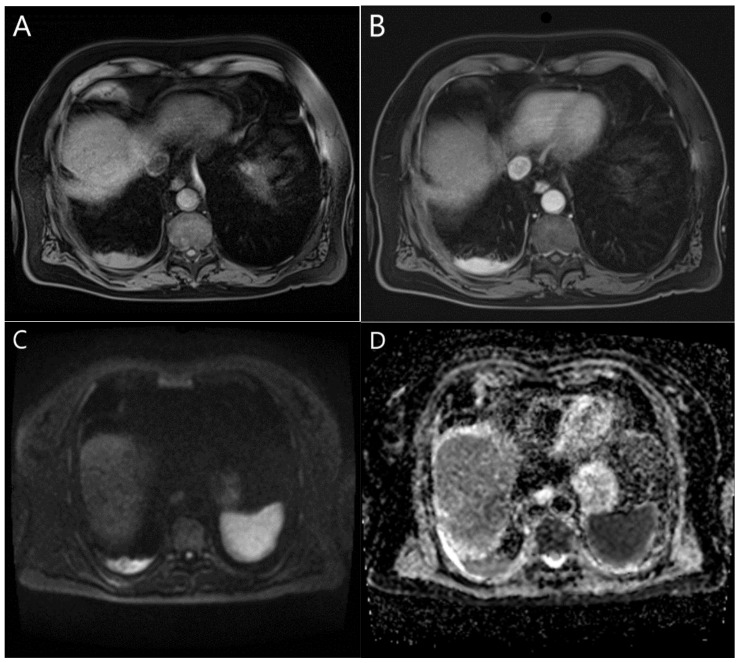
MR images indicate thickening/consolidation within the dorsal aspect of right pleural cavity, consistent with mesothelioma. Pre- (A) and post-contrast (B) T1-weighted vibe axial images with fat saturation depict vividly enhancing lesion, with apparent signs of diffusion restriction on high b-value (b = 800) DWI images (C) and complimentary ADC maps (D).
